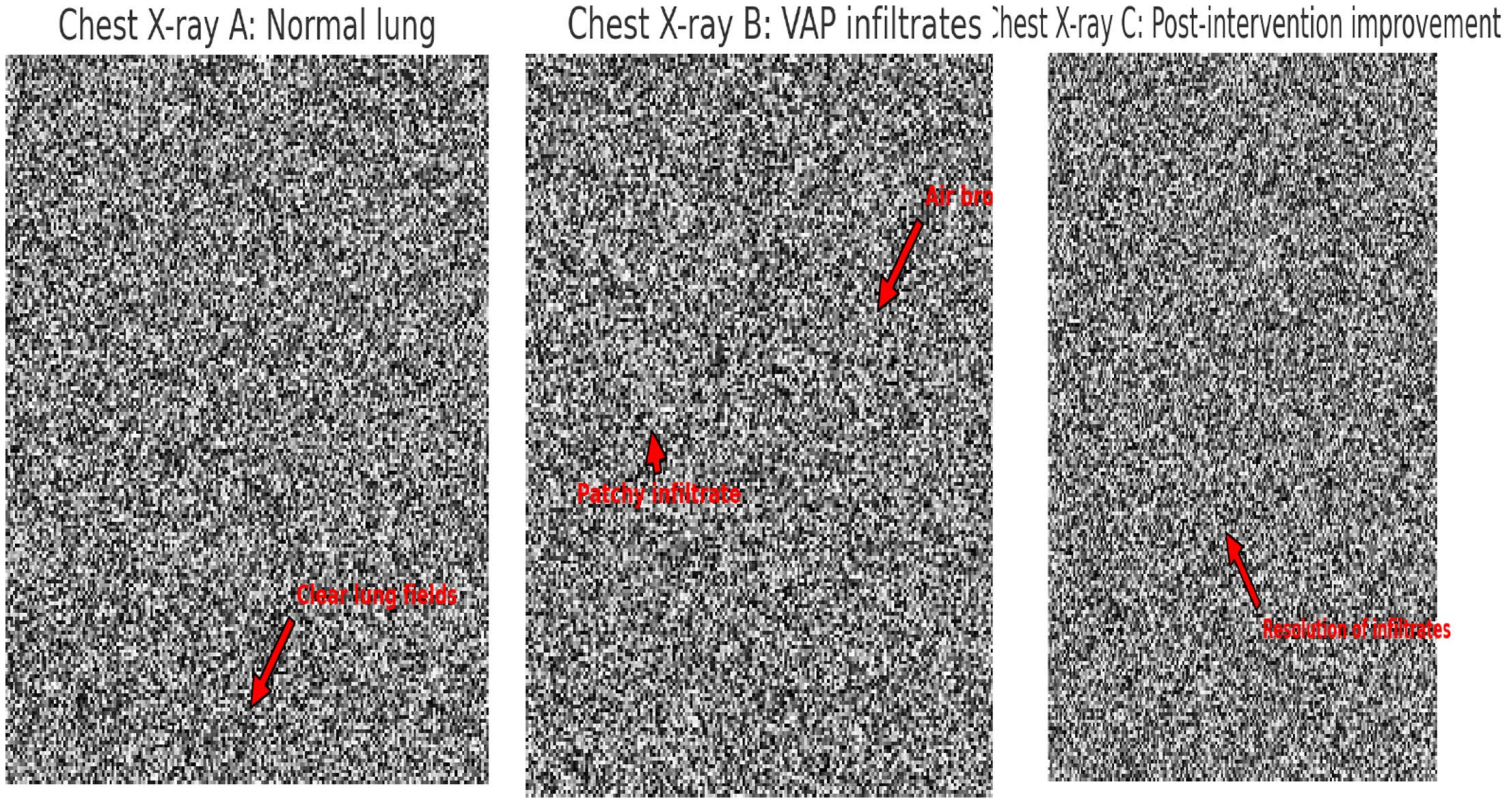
Chest X-rays. (A) Normal lung fields on mechanical ventilation. (B) Classic radiographic signs of VAP. (C) Post-intervention image.
